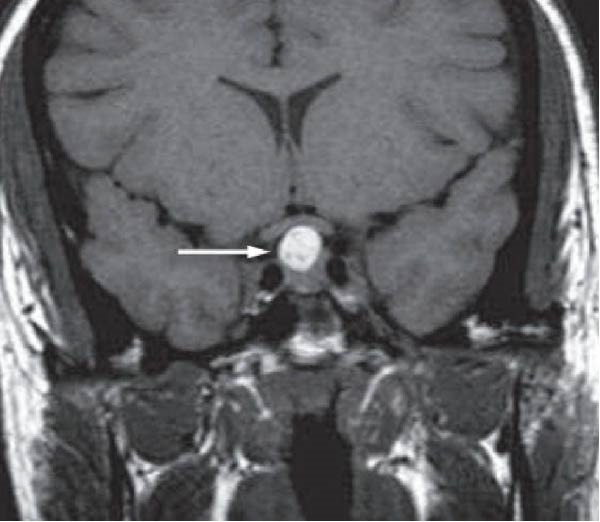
Brain MRI coronal section showing a well‐defined lobulated mass measuring 6.3 cm × 2.0 cm ×1.7 cm in the sella turcica compressing the optic chiasma.
